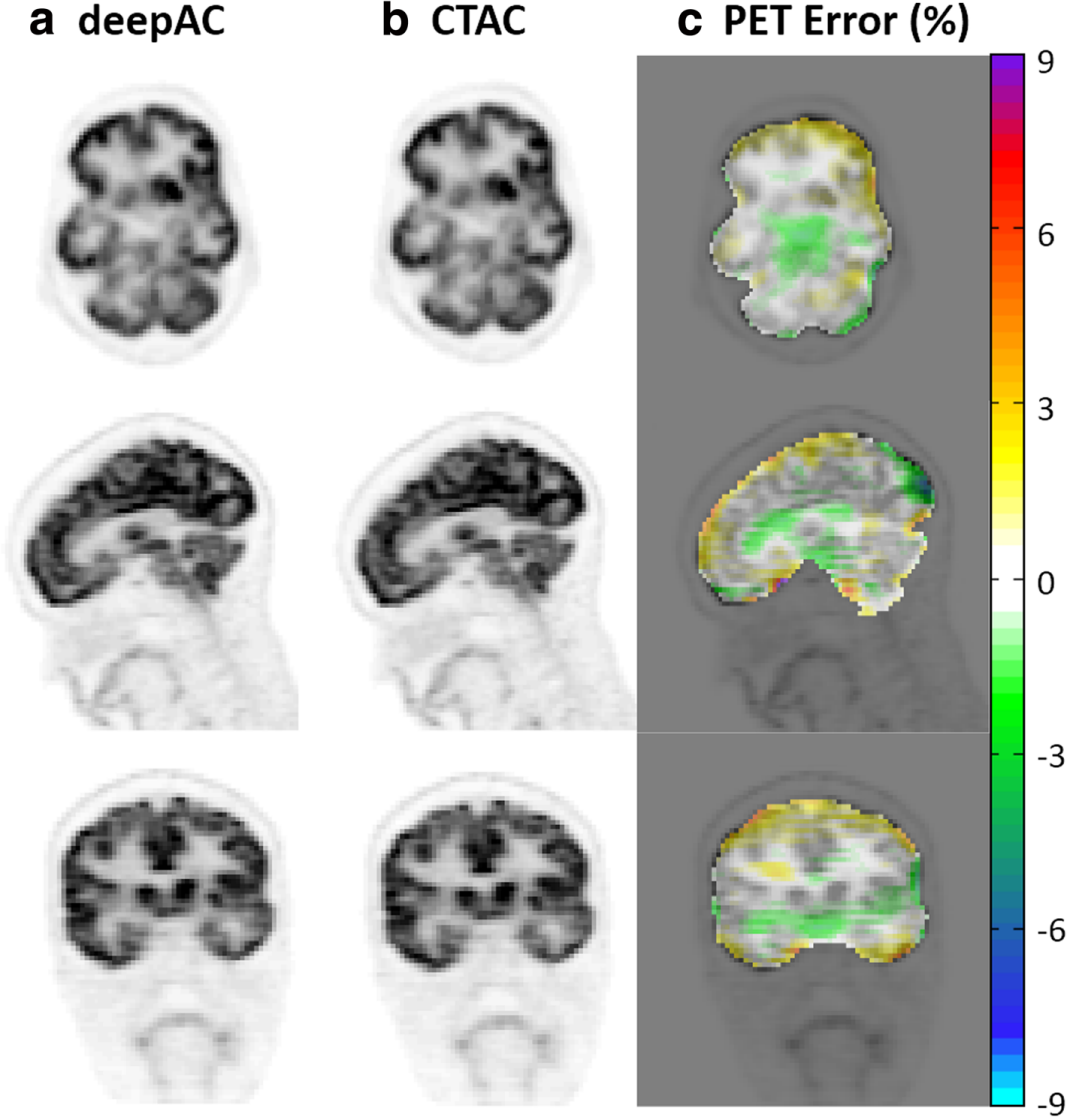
PET reconstruction using a deepAC and b acquired CT-based attenuation correction (CTAC) for a 48-year-old female subject. c Relative error was calculated using the PET image reconstructed using CTAC. Low reconstructed PET error is observed by using the proposed deepAC approach
